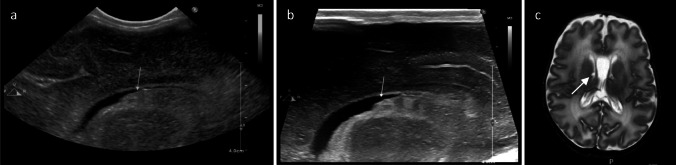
Intraventricular haemorrhage (IVH) grade I in a 29-week-gestation newborn, 10 days of life. a, b Right parasagittal scans. The definition of the subacute clot (inhomogeneous hyperechoic lesion on the ventricular floor, arrow) is lower using the micro-convex transducer (a) compared to the very high-frequency transducer (b). MRI performed at term age (c): the IVH is no longer visible, while a germinolytic cyst is detected (arrow)
